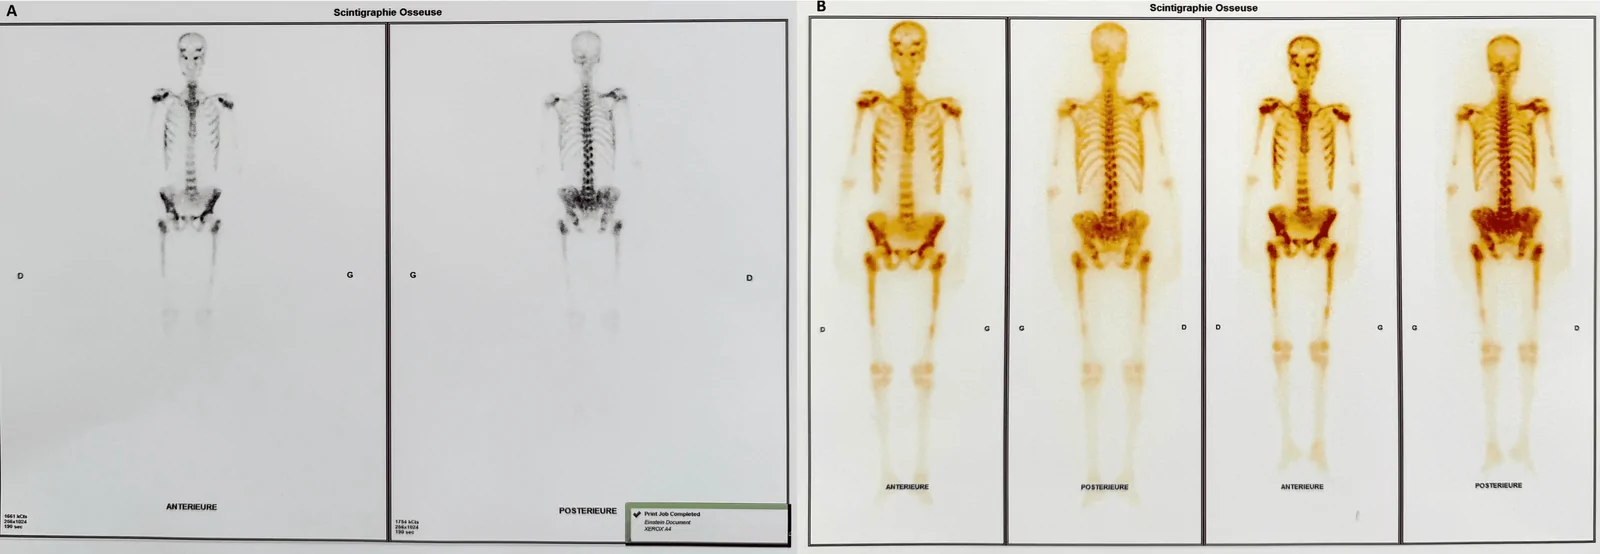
Whole-body bone scintigraphy demonstrating a metastatic super scan. (A) Anterior and posterior planar scintigraphic images. (B) Color-rendered scintigraphic images.
